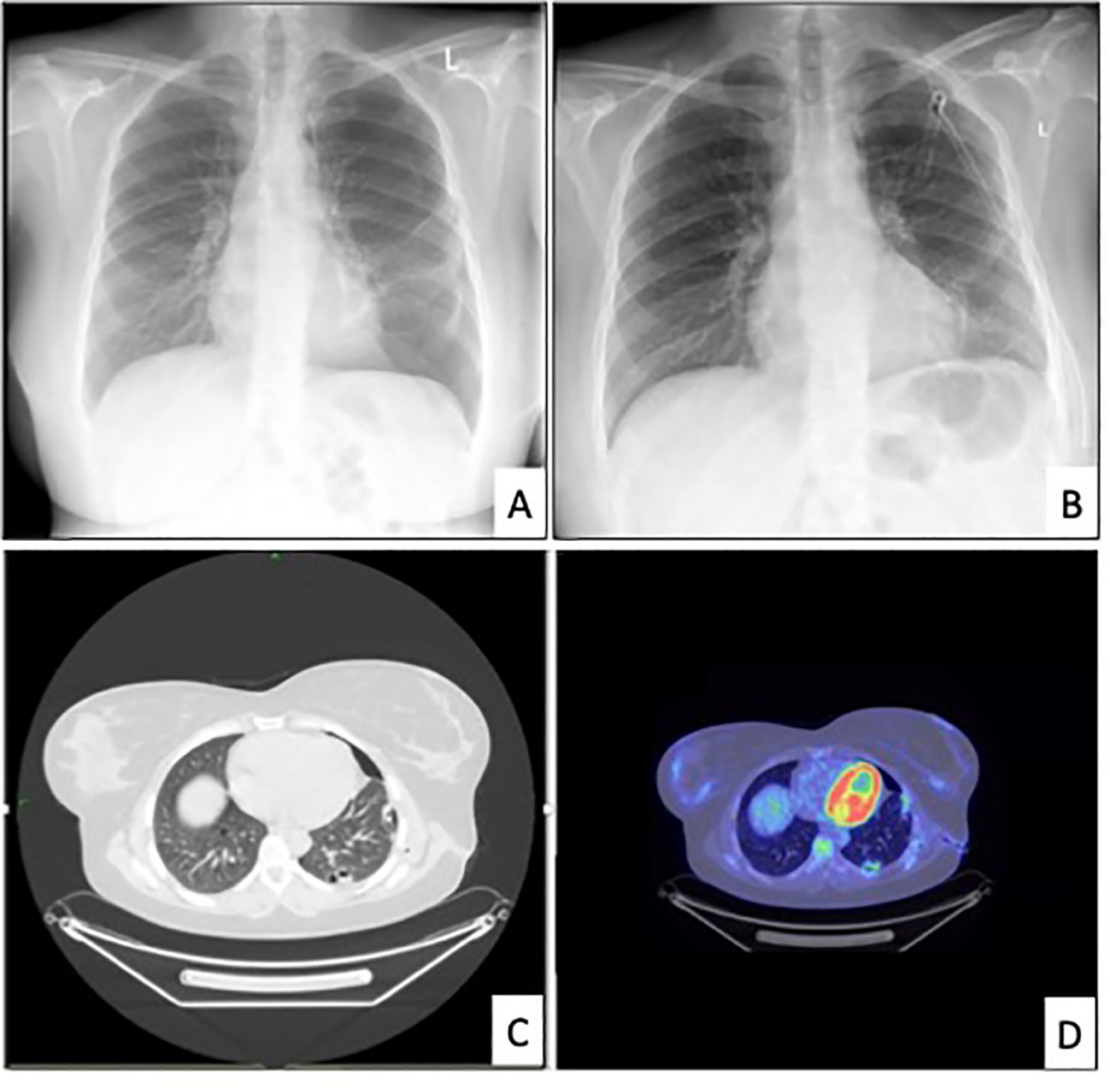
Relevant radiological images. [(A) chest x-ray revealing the recurrence of pneumothorax in June 2022. (B) chest x-ray after drainage, (C) chest CT in June 2022, (D) PET-CT in July 2022].
